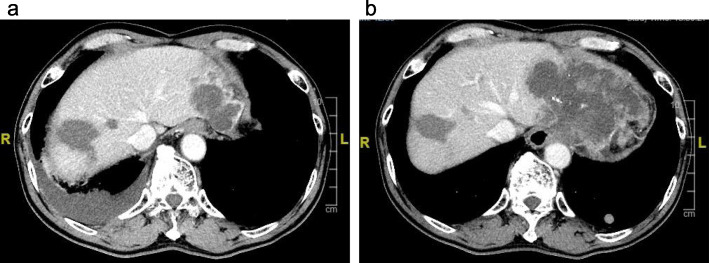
Contrast-enhanced computed tomography (CECT). CECT revealed multiple hepatocellular carcinoma (HCC) recurrences in the lateral segment (maximum size, ~ 4.0 × 3.0 cm) (a). The recurrent HCC enlarged quite rapidly (size 13.0 × 8.0 cm) and showed ringlike contour enhancement and central necrosis (b)
